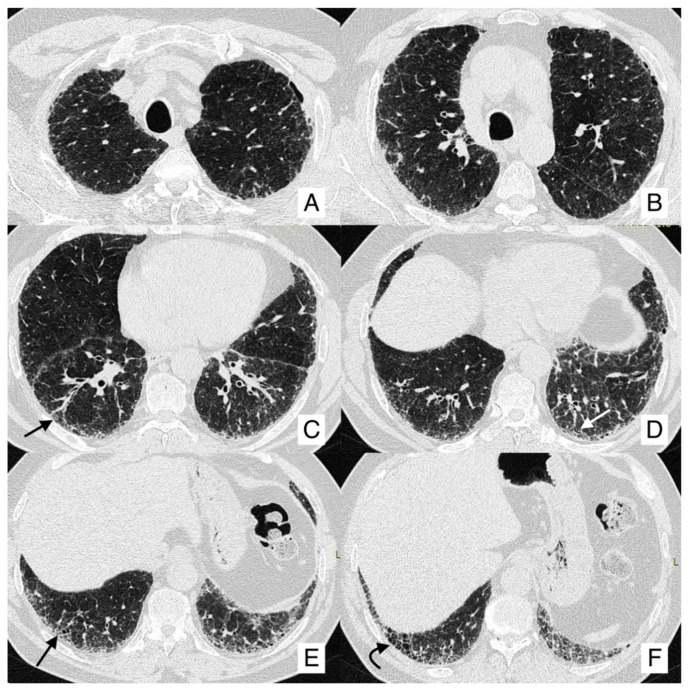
A patient with typical HRCT features of IPF. Images acquired at three different levels of the thorax (A,B) obtained above the carena; (C,D) scans obtained at middle levels of lungs; (E,F) acquired at basal regions. Reticulations are located in peripheral regions, more represented in the medium regions of the lung, and at the pulmonary basis (black arrows); there is a typical cranio-caudal gradient of these imaging findings. Cystic spaces are well-demonstrated (honeycomb area is clearly recognizable in the right costophrenic angle, black curved arrow in (E), reticulations and small bronchiectasis are well-appreciated (black arrows in (C,E), white arrow in (D). Therefore, a UIP pattern could be recognized.
